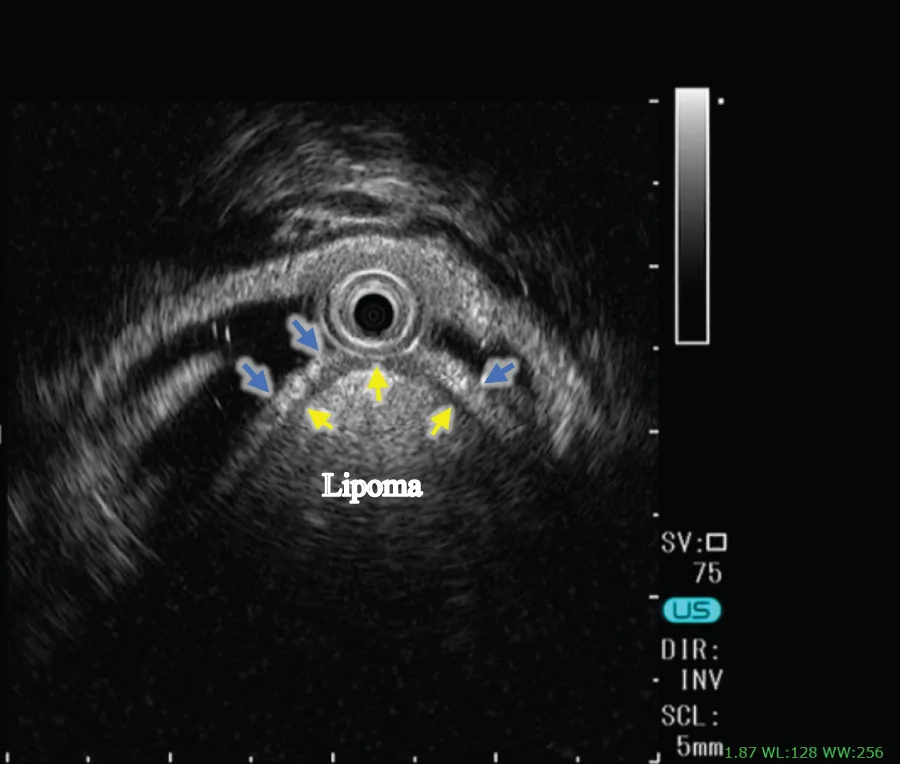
EUS findings of the tumor and lipoma. Blue arrows: Superficial mucosa (hyperechoic area). Yellow arrows: Deep mucosa (hypoechoic area). Lipoma: A homogeneous hyperechoic area was observed in the 3rd layer, which was consistent with a lipoma. EUS, endoscopic ultrasonography
